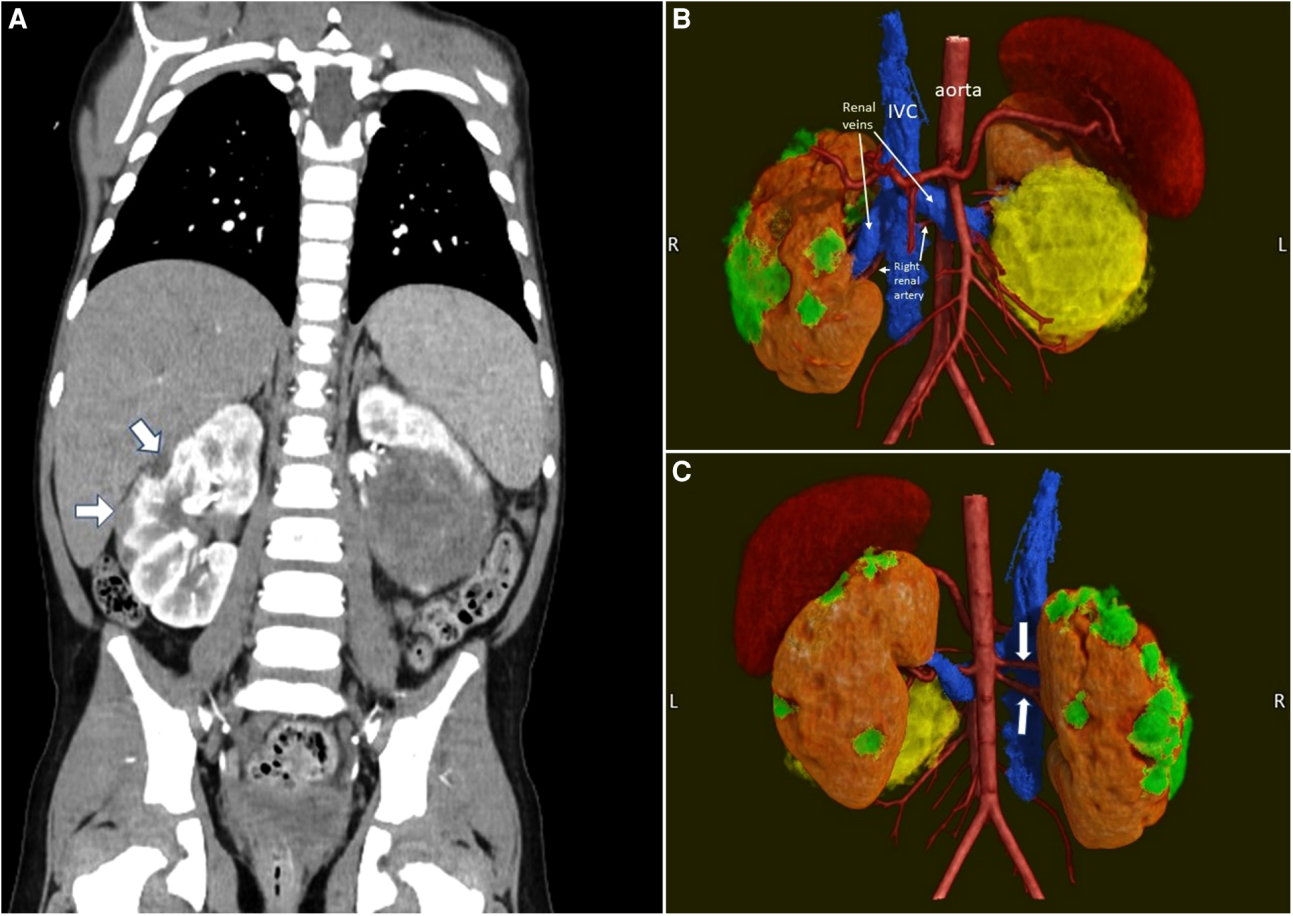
Pre-operative imaging of a case of synchronous bilateral Wilms tumor. (A) Pre-operative CT scan. Note the multiple peripheral lesions on the right kidney. (B) 3D rendering (anterior view) of the kidneys, their tumors and the vasculature. The smaller right kidney tumors are in green. The large left kidney tumor is in yellow. (C) 3D rendering (posterior view). Note the two right renal arteries (white arrows).
